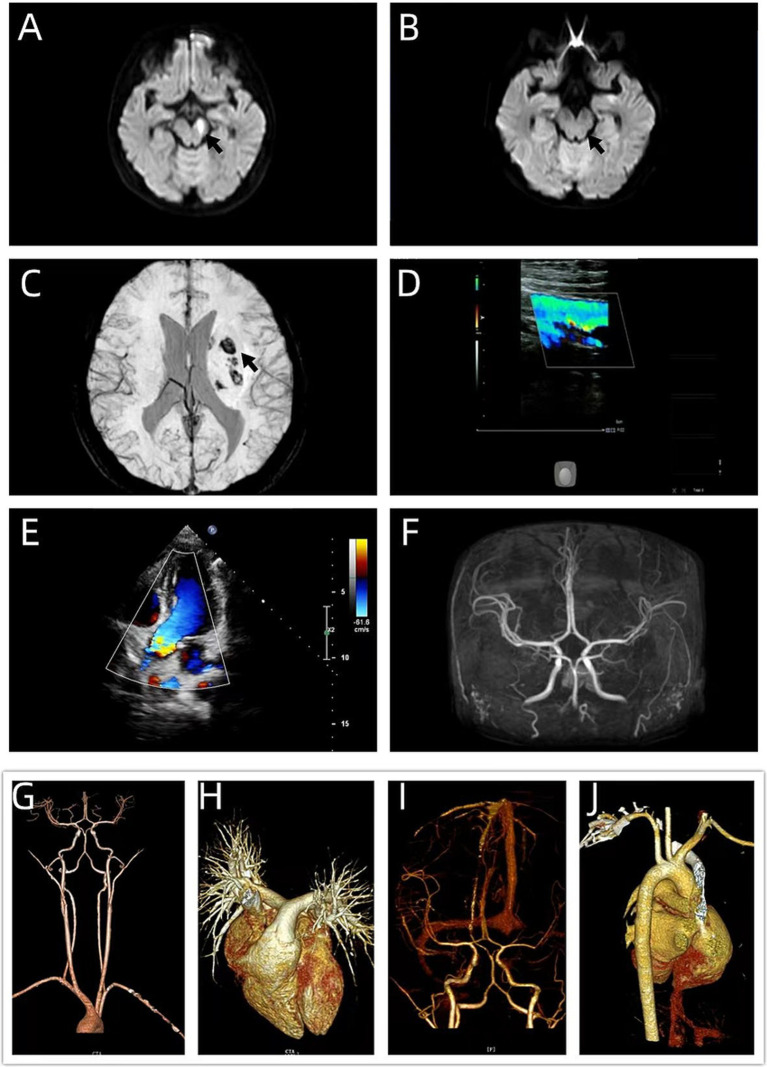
Follow-up imaging and systemic evaluation for embolism. (A,B) Comparison of brain DWI sequences. (A) (Current study) shows a new patchy hyperintensity in the left cerebral peduncle, consistent with acute cerebral infarction (arrow). (B) (Study from 14 days prior) shows no abnormal signal in the corresponding region (arrow). (C) Follow-up DWI and susceptibility-weighted imaging (SWI) reveals decreased DWI signal in the original left basal ganglia-periventricular infarction. SWI shows punctate hypointense foci within the lesion (arrow), consistent with subacute infarction accompanied by hemorrhagic transformation. (D,E) Embolism workup. (D) (Color Doppler ultrasound of the extremities) and (E) (Contrast transthoracic echocardiography) show no definitive thrombotic source or right-to-left shunt (RLS grade 0). (F–J) Systemic vascular imaging evaluation. Brain MRA, neck CTA, pulmonary artery CTA, pulmonary vein and left atrium CTA, intracranial CTA, and ascending aorta CTA show no evidence of arterial stenosis, dissection, or embolism.
